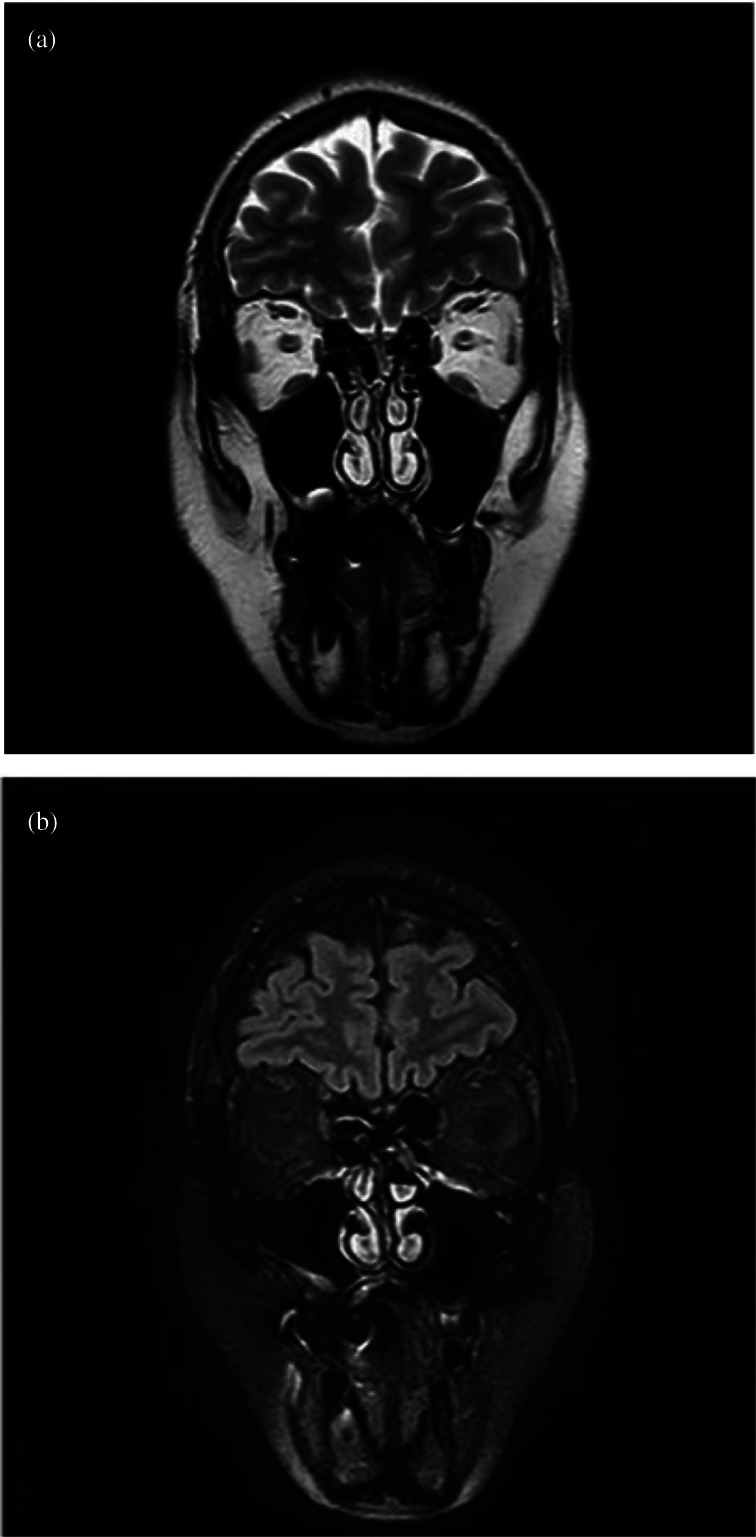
Magnetic resonance imaging did not reveal any pathological findings: the olfactory bulb and clefts were of normal volume, without signal anomalies. Coronal scans of: (a) T2-weighted fast spin echo sequence, and (b) T2-weighted fluid-attended inversion recovery with fat suppression sequence.
